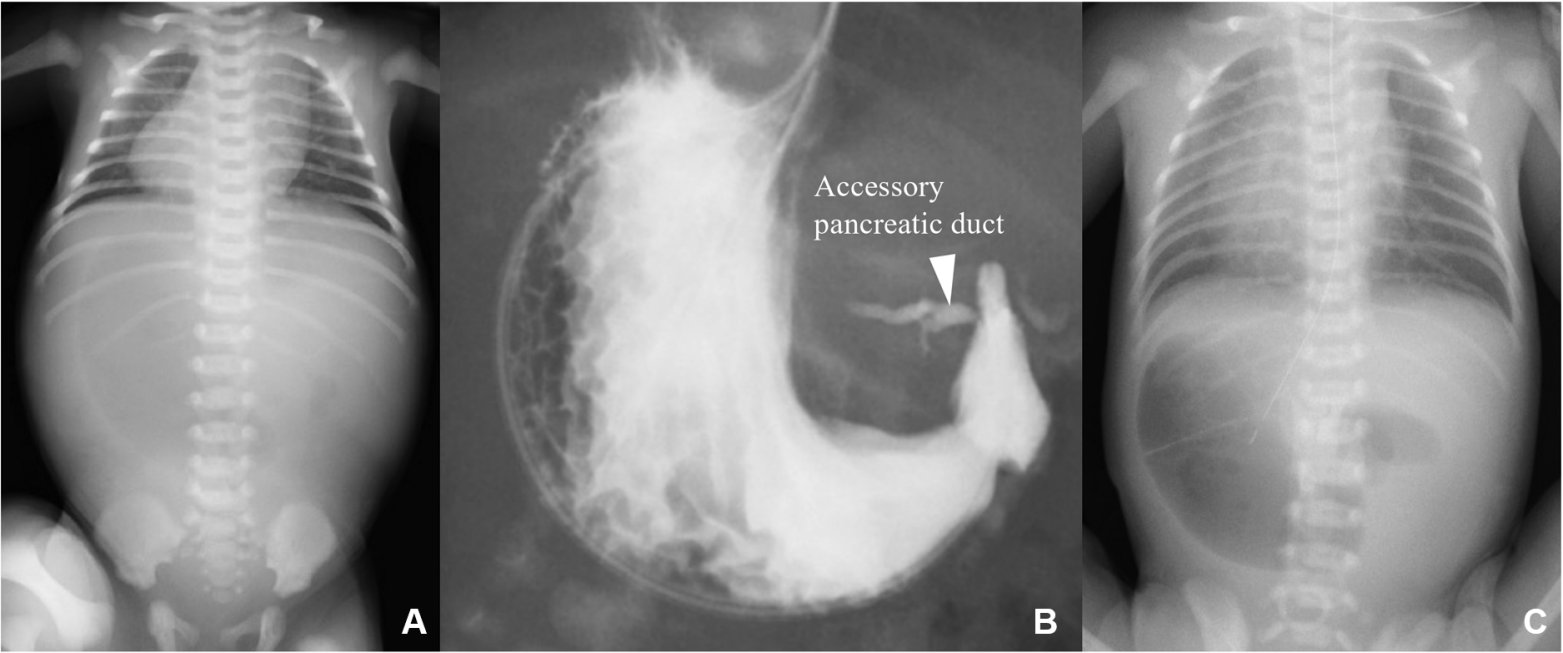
Preoperative imaging studies. (A) Chest radiograph showing the double-bubble sign with situs inversus. (B) Upper gastrointestinal contrast-enhanced imaging revealing duodenal atresia. The distal duodenum was enhanced through the accessory pancreatic duct. (C) Abdominal radiograph showing the double-bubble sign with situs inversus.
